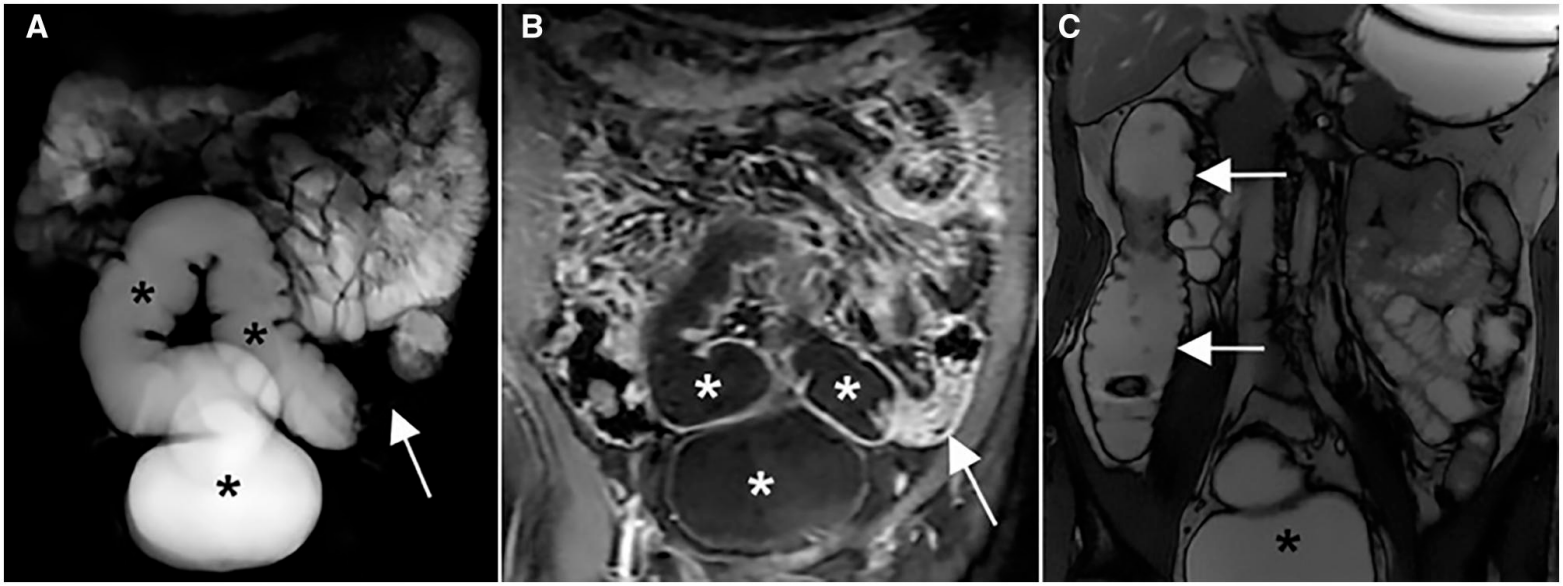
Hydro-MRI in a patient with known Crohn’s disease. Coronal single-shot fast spin-echo (A) and T1-weighted after gadolinium injection (B) images show good distension of rectum and sigmoid colon after rectal enema (asterisks); a stenosis is evident in the proximal sigmoid colon (white arrow); Coronal SSFP Fid + Echo image (C) after rectal enema (black asterisk) demonstrates a good distension of right colon (white arrows).
